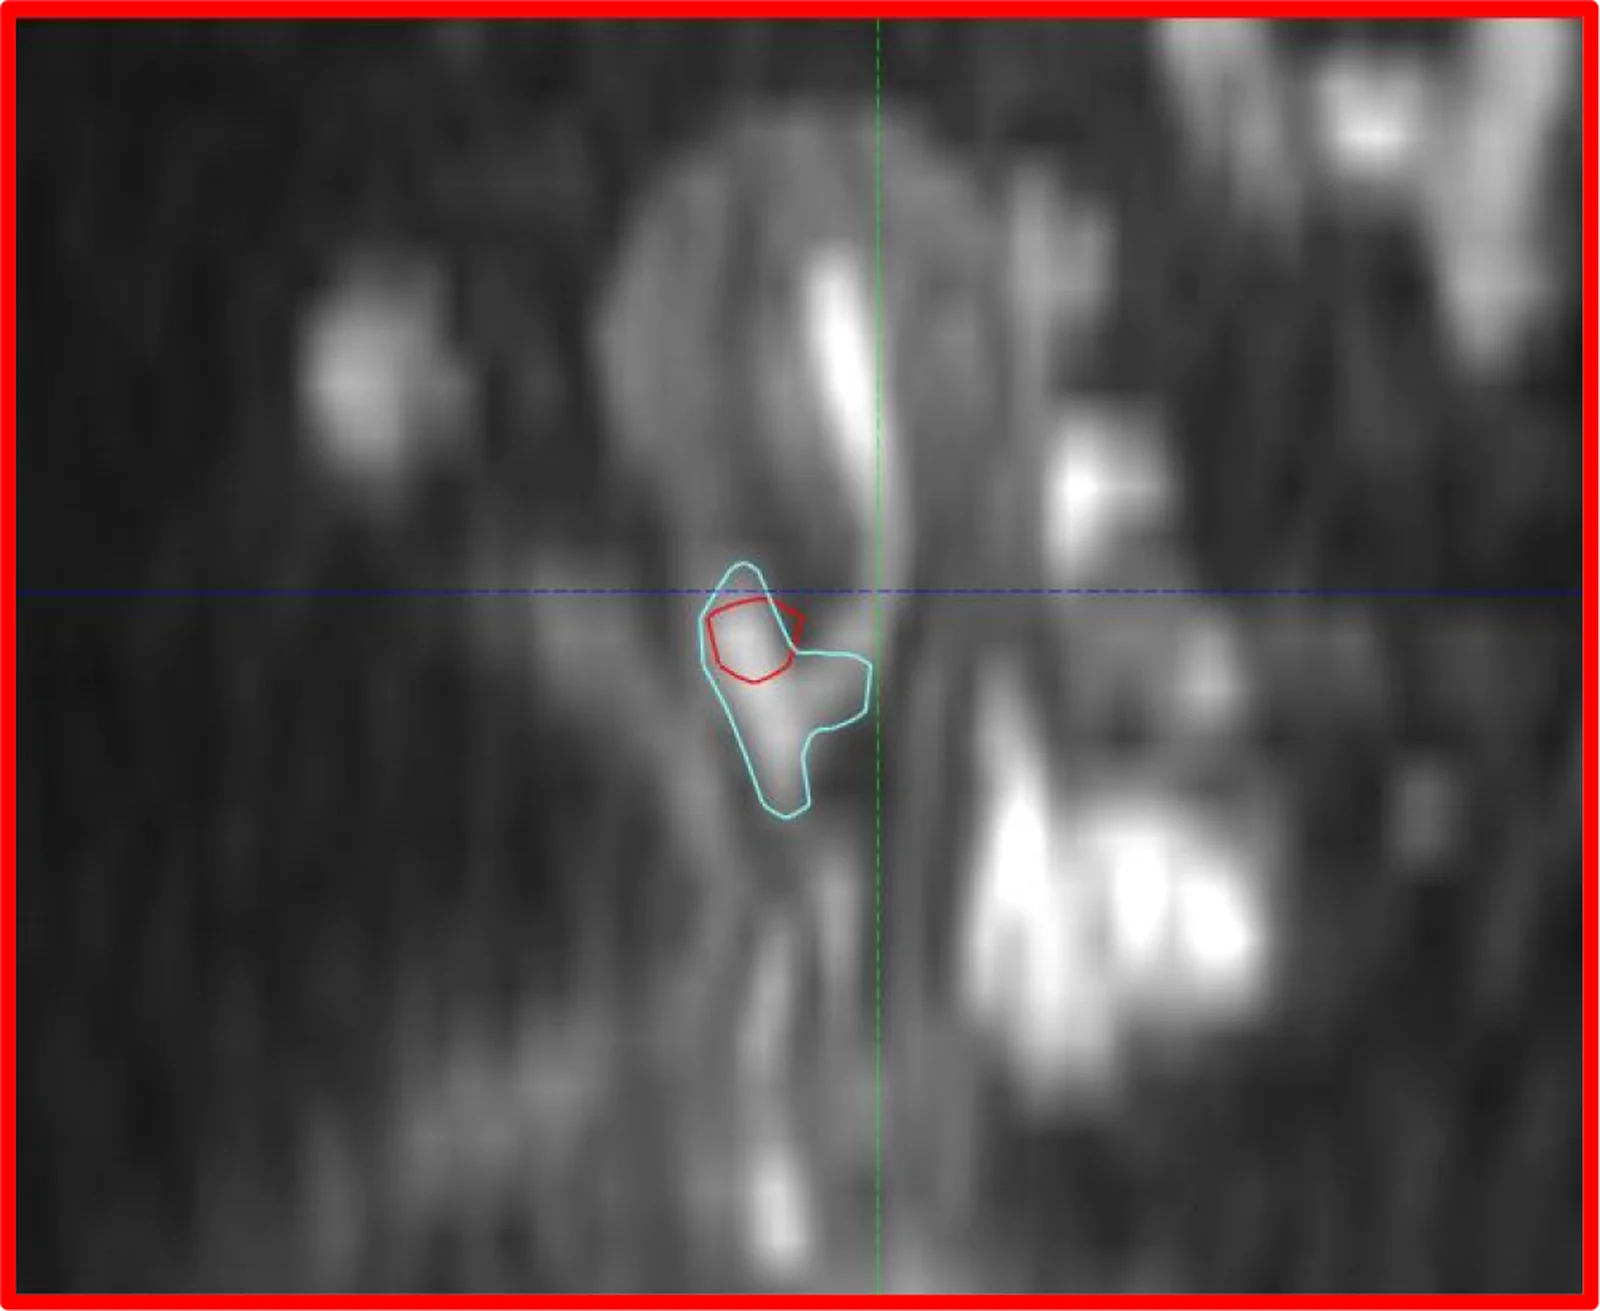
a. Figure showing GTV_T2 at pre-brachytherapy on T2 weighted MRI, coronal view. b. Figures showing GTV_T2/DWI at pre-brachytherapy on DWI in cyan outline with the corresponding GTV_T2 in red outline, coronal view. (For interpretation of the references to colour in this figure legend, the reader is referred to the web version of this article.) c. Figure showing GTV_T2 at pre-brachytherapy on T2 weighted MRI, sagittal view. d. Figures showing GTV_T2/DWI at pre-brachytherapy on DWI in cyan outline with the corresponding GTV_T2 in red outline, sagittal view. (For interpretation of the references to colour in this figure legend, the reader is referred to the web version of this article.)
